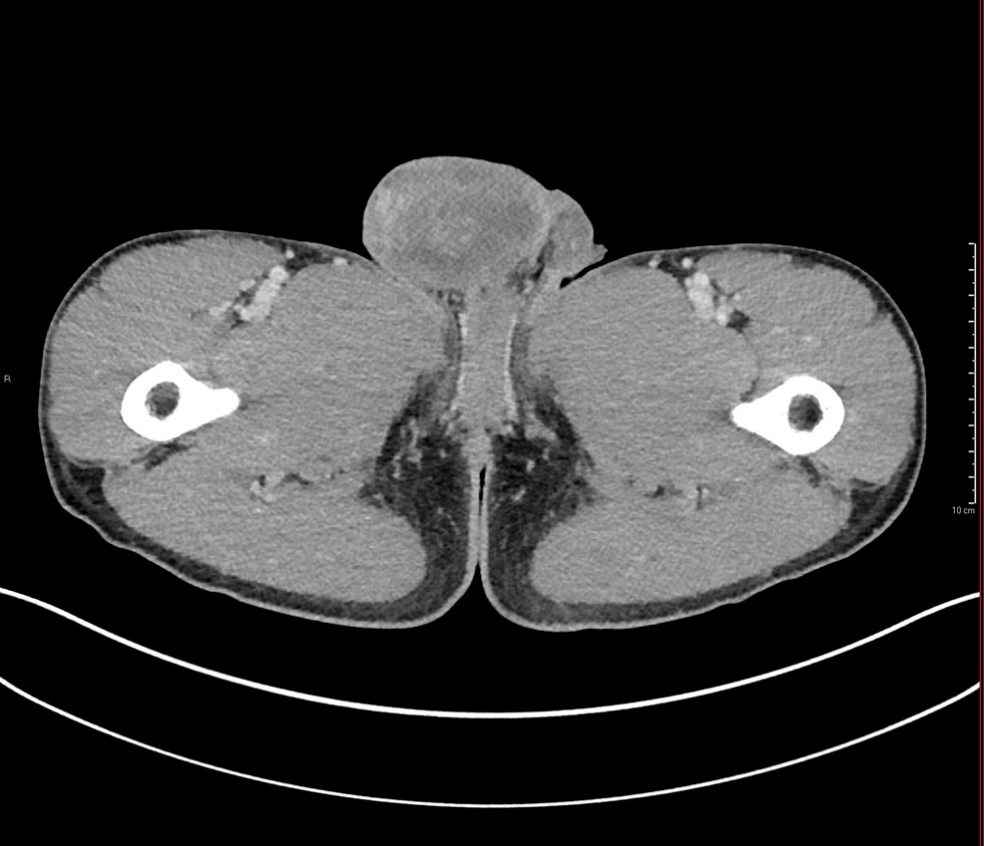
Axial intravenous contrast-enhanced computed tomography image of the abdomen and pelvis demonstrating a heterogeneous right testicular mass
